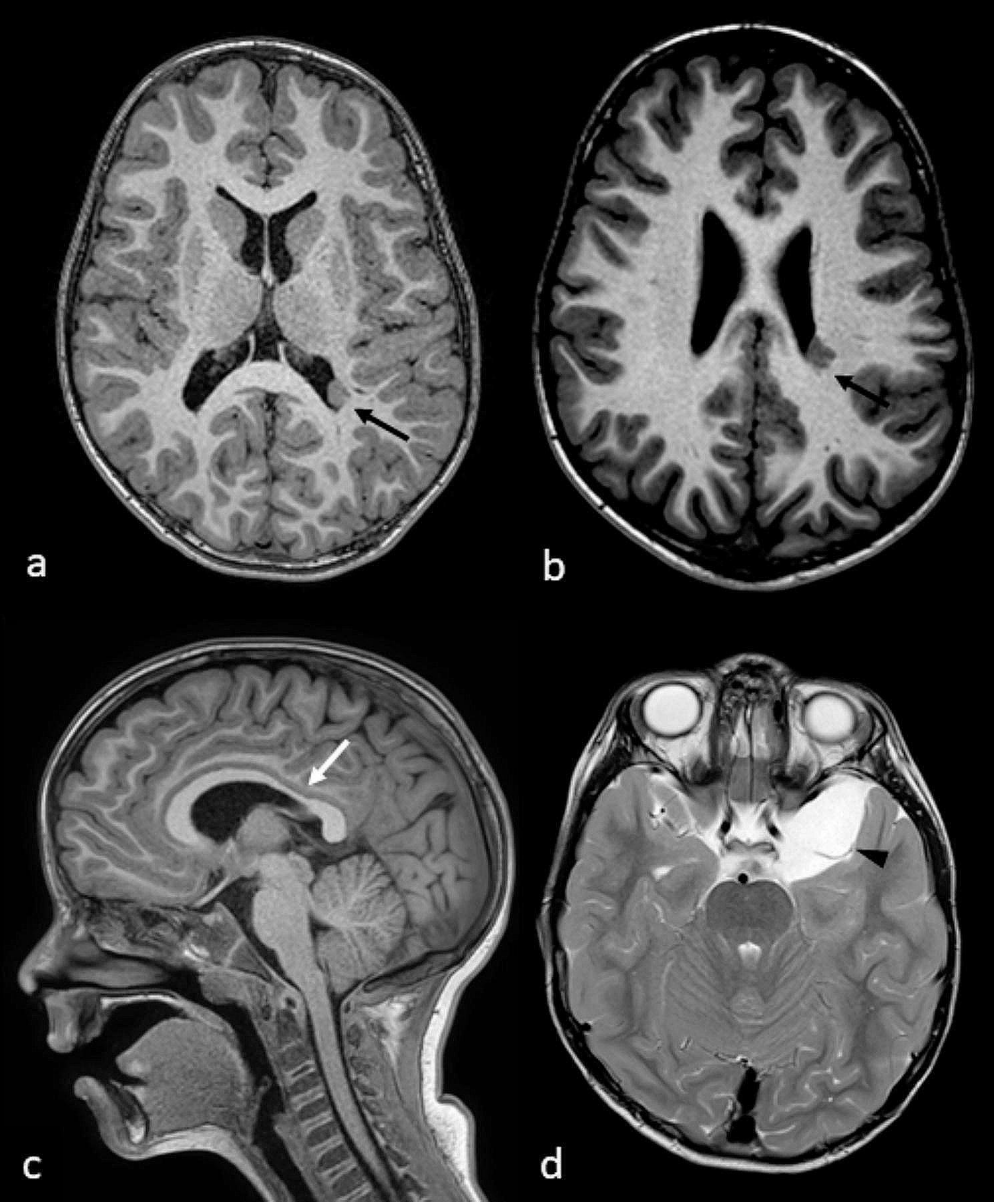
Brain MRI (6y 11 m), axial T1 (a-b), midline sagittal T1 (c) and axial T2 (d): periventricular nodular heterotopia in the posterior horn (a, black arrow) and middle portion (*b*, black arrow) of the left lateral ventricle, mild thinning of the corpus callosum istmo (*c*, white arrow), and arachnoid cyst in left mesial temporal lobe (*d*, arrowheads)
